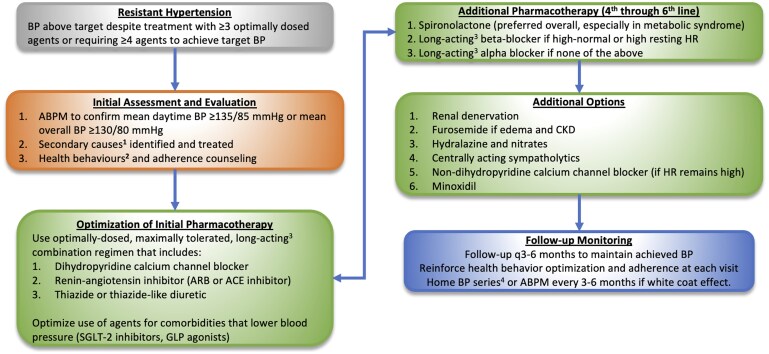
Algorithm for the assessment and management of resistant hypertension. ^1^Screen all patients for primary aldosteronism (plasma aldosterone/renin ratio), drug and alcohol use disorder, renal parenchymal disease (urinalysis, serum creatinine, ultrasound) and selected patients (based on symptoms and signs) for obstructive sleep apnea (Epworth Scale, overnight polysomnography), renal artery stenosis (CT or MR angiography), pheochromocytoma (plasma or 24-h urinary metanephrines), Cushing’s syndrome (24-h urinary free cortisol, late-night salivary cortisol and/or 1 mg dexamethasone suppression test), hyper/hypothyroidism (TSH), aortic coarctation (thoracic CT or MRI), primary hyperparathyroidism (serum calcium). ^2^Sodium restriction to 2 g per day or less, weight loss to target BMI <25 kg/m^2^, DASH diet, 30-60 min exercise on most days of week, limit excessive alcohol intake, smoking cessation, sleep optimization, stress management. ^3^Long-acting agents include: CCB (amlodipine, long-acting nifedipine), ACE inhibitor (perindopril, trandolapril), ARB (telmisartan, olmesartan, irbesartan), beta-blocker (bisoprolol, long-acting metoprolol), alpha blocker (doxazosin). ^4^Mean of duplicate readings performed in morning and evening for 7 days.
